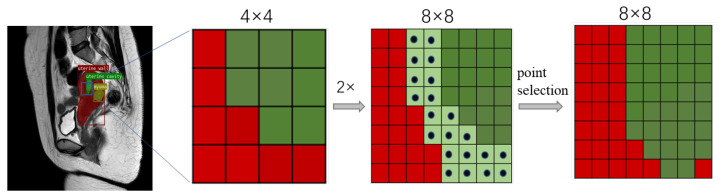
Adaptive iterative subdivision step of edge points on a uterine MR image. First, rough predictions are performed on a 4 × 4 grid, and bilinear interpolation is used to upsample twiceon the prediction. After that, the 21 most ambiguous points are selected on a finer, 8 × 8 grid. After PointHead’s prediction, detailed pointwise features are recovered. This process is repeated until the segmentation is upsampled to the desired spatial resolution.
